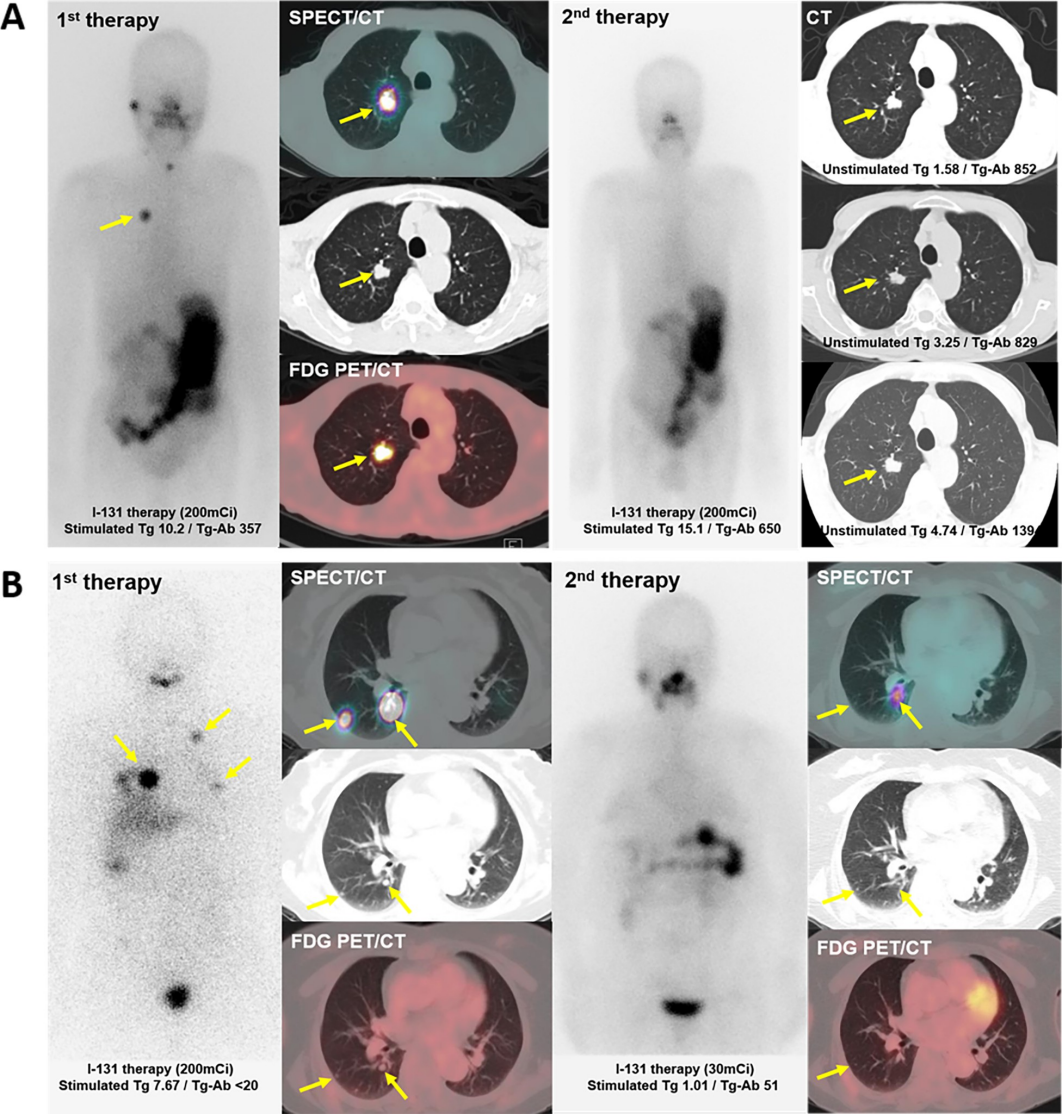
The representative cases showing the predictive role of FDG uptake in RAI therapy. A. The patient who has lung metastasis from papillary thyroid cancer was done RAI therapy after total thyroidectomy. After first therapy, there was quite amount of RAI in the metastatic lesion, expecting therapeutic effect of RAI. However, initial FDG PET scan showed there are also substantial FDG uptake in the metastasis. At the time of 2^nd^ therapy, the RAI uptake was gone and the anatomical size of lung metastasis was not changed during serial follow-up CT scan. Even the unstimulated Tg level as well as stimulated Tg level was subsequently increased. B. The patient who has recurred metastatic lung nodules from papillary thyroid cancer after total thyroidectomy 10 years ago. The metastatic nodules had a significant amount of RAI uptake in the post-therapy scan, but did not show significant uptake in the FDG PET scan performed at the same time. At the time of 2^nd^ therapy, the size of lesions was significantly decreased and stimulated Tg level was also lowered.
